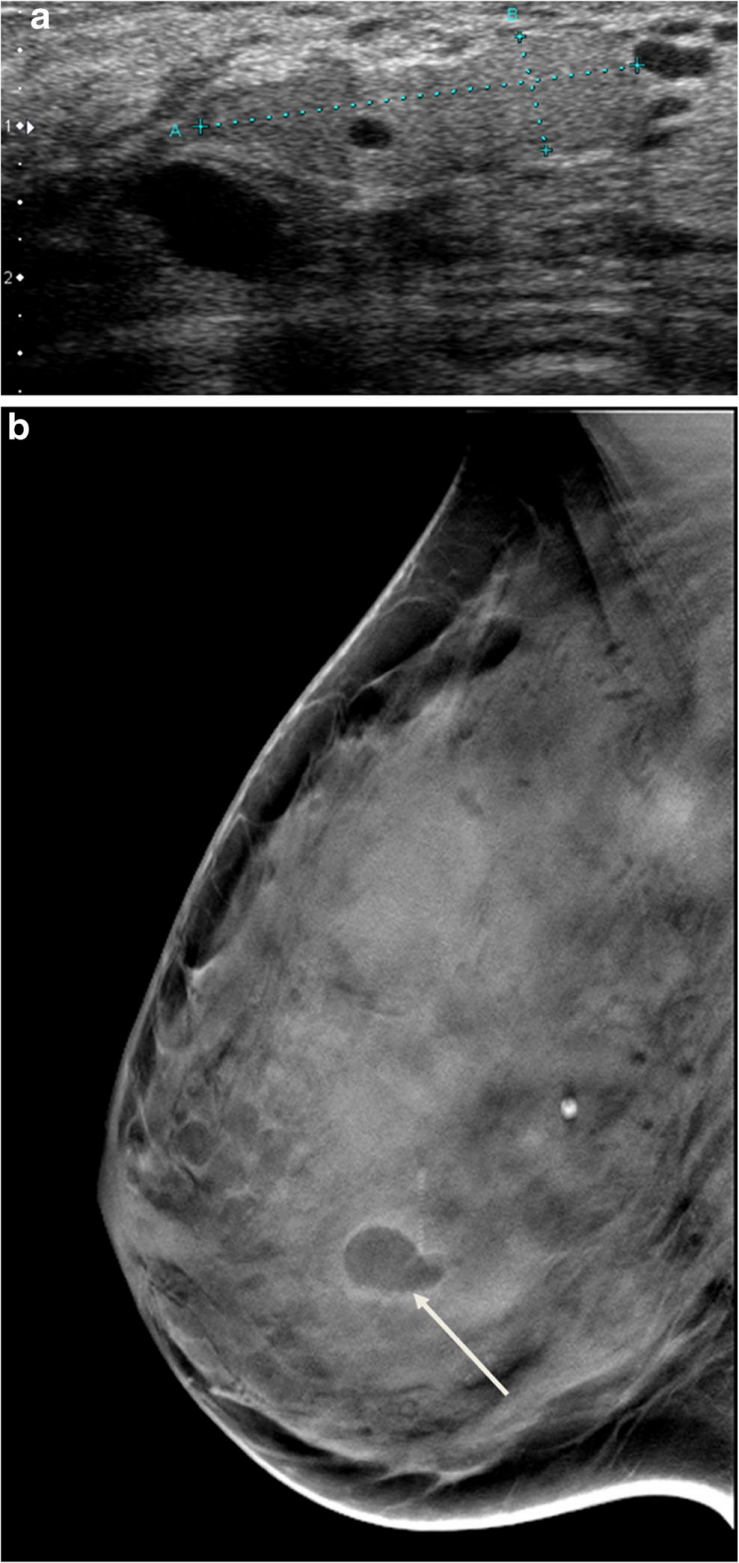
Galactocele. a Ultrasound scan showing duct dilatation with homogeneous hyperechoic content, with no blood supply demonstrated by colour Doppler, in a schizophrenic patient with long-term neuroleptic treatment. b Digital breast tomosynthesis in the same patient showing a fat density galactocele (*arrow*)
